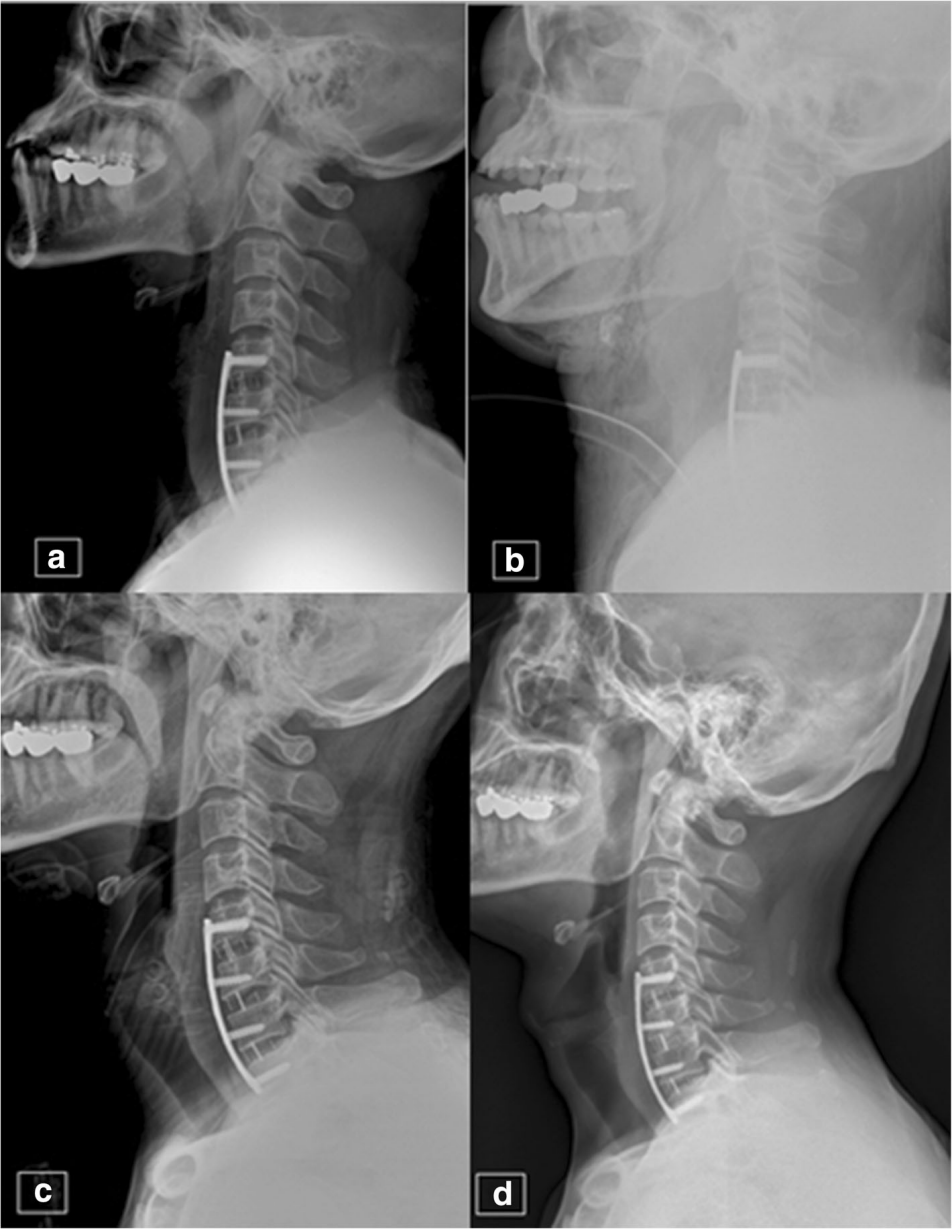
A 47-year-old male patient. a Lateral radiograph of the cervical spine on the day of surgery showing anterior cervical discectomy and fusion at C5-6, C6-7, and C7-T1. b Lateral radiograph at 10 h postoperatively during adult respiratory failure showing severe prevertebral swelling and cricothyrotomy. The patient had cyanosis and respiration was not responsive. Oxygen saturation was measured at 60–70%. c Lateral radiograph at 17 days postoperatively showing decreased prevertebral swelling. The patient was discharged without complications. d Lateral radiograph at the 12-month follow-up showing no prevertebral swelling
